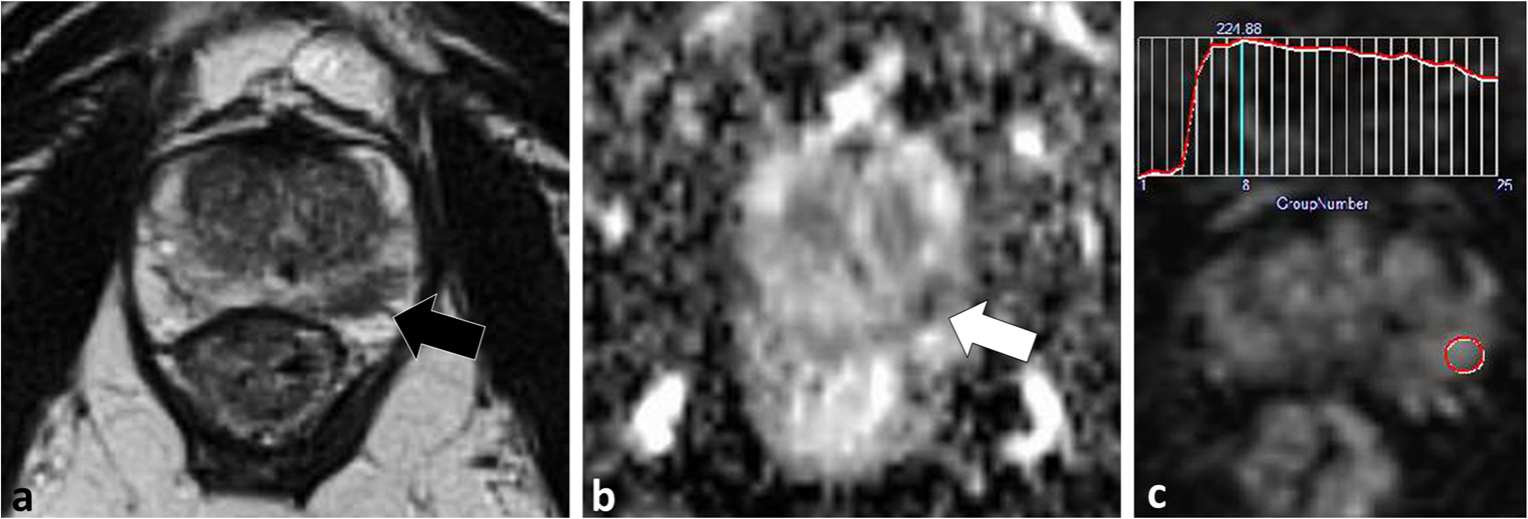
A 63-year-old patient underwent MP-MRI prior to routine repeat biopsies as part of his active surveillance protocol. Axial T2W TSE (a), axial ADC map (b) and semi-quantitative contrast curve derived from DCE (c) demonstrate a focal low T2W SI lesion (*black arrow*) with restricted diffusion (*white arrow*) and type III kinetics in the left middle peripheral zone. At repeat targeted biopsy using cognitive registration, no corresponding lesion could be identified. With a priori knowledge of the location of the lesion at MP-MRI, the TRUS operator performed three core needle biopsies through the left middle lateral and two core needle biopsies through the left middle medial PZ sextants. Results after targeted biopsies were Gleason 4 + 3 = 7 tumour with two out of three core biopsies in the left middle lateral PZ sextant
